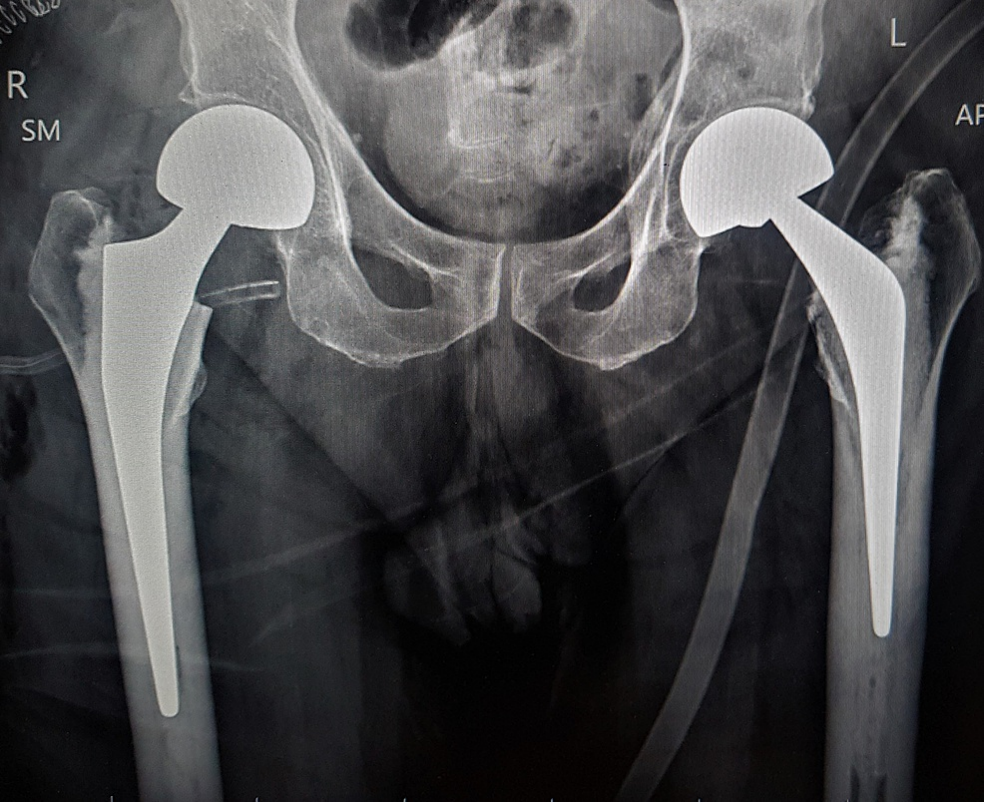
Pelvis radiograph with both hips' bipolar prosthesis in situ
